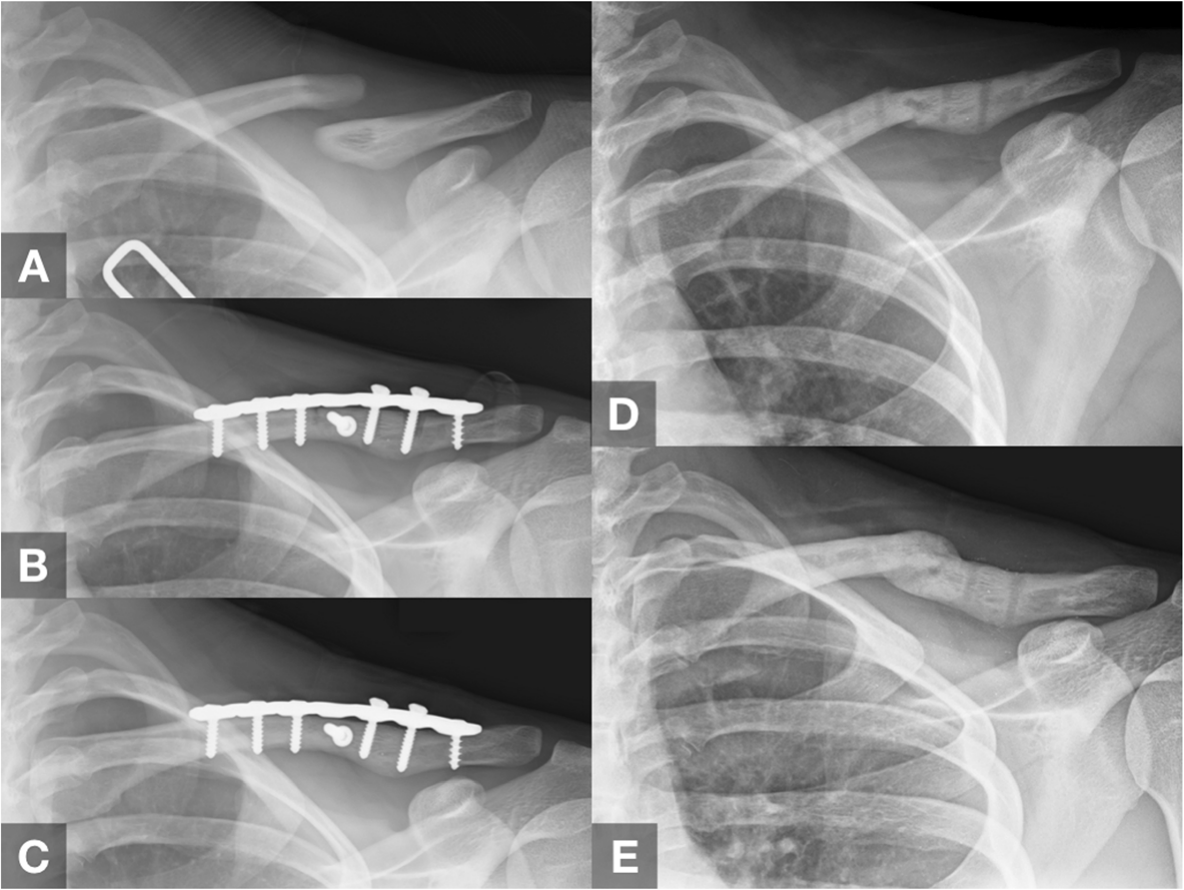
Case demonstration of a 25-year-old female patient. a Preoperative anteroposterior radiograph of the clavicle. b Immediately after open reduction and plate fixation surgery. c Twelve months after fixation surgery, before plate removal. d Refracture occurred 4 weeks after plate removal. e Conservative treatment for 3 months with solid radiographic union
